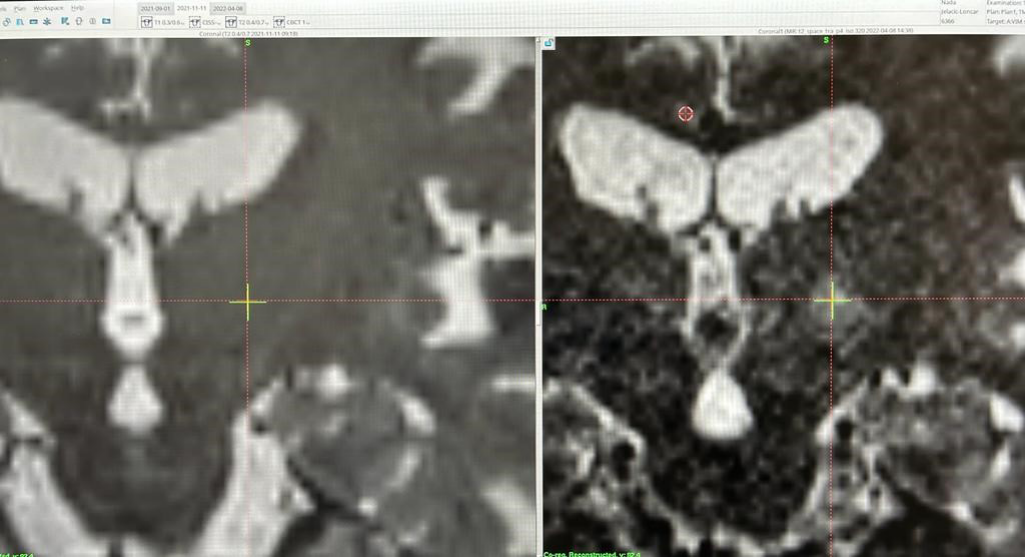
A brain magnetic resonance image (MRI) of case 2 comparing a baseline MRI coronal T2 sequence (left) to a control MRI coronal T2 sequence (right) done eight months after gamma knife thalamotomy.
